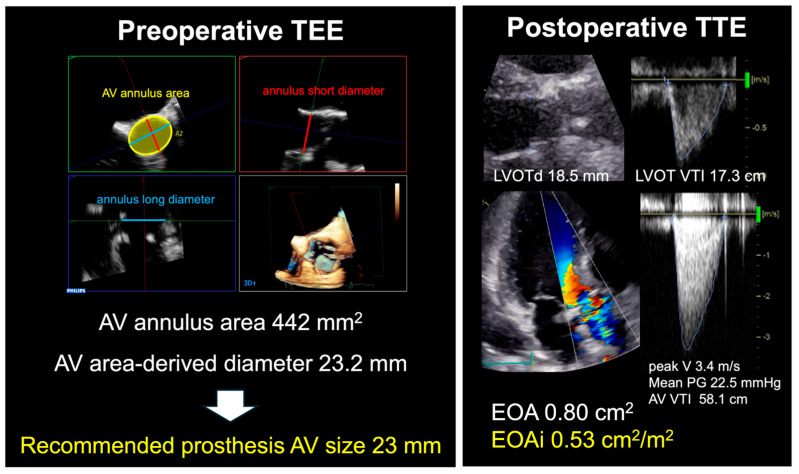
Representative case of severe postoperative prosthesis-patient mismatch (PPM). The patient was a 68-year-old female with aortic stenosis. The aortic valve (AV) annulus area was 442 mm^2^ (yellow circle) and the AV annulus area-derived diameter was calculated as 23.2 mm. This patient was implanted with a 21-mm prosthetic valve, which was smaller than the recommended prosthesis AV size. AV annulus diameter was reduced by 9.5% after the procedure and severe PPM occurred. TEE; transesophageal echocardiography, AV; aortic valve, TTE; transthoracic echocardiography, V; velocity, PG; pressure gradient, EOAi; effective orifice area index.
